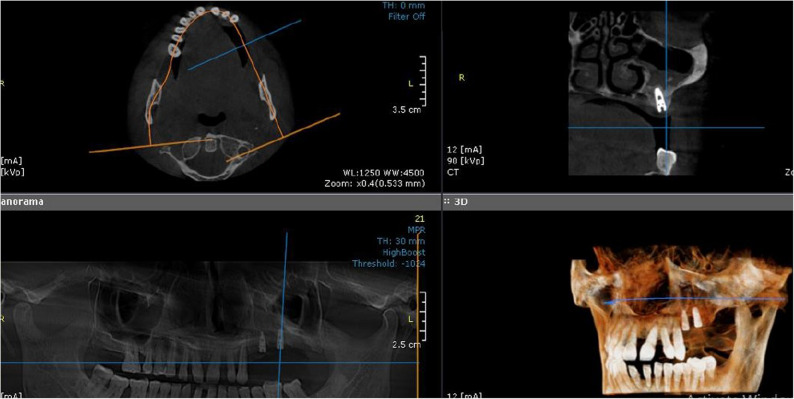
CBCT image after implant placement demonstrating osseointegration. Postoperative CBCT scan shows successful implant placement in the augmented maxillary area, with evidence of proper osseointegration and stable bone surrounding the implant
